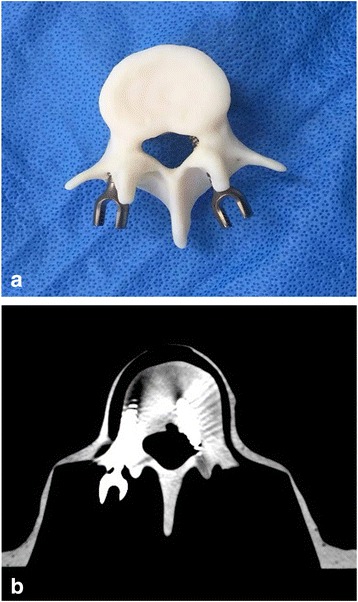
a The spinal models underwent b CT scan immediately after the pedicle screws were inserted to evaluate their instrumentation accuracy
